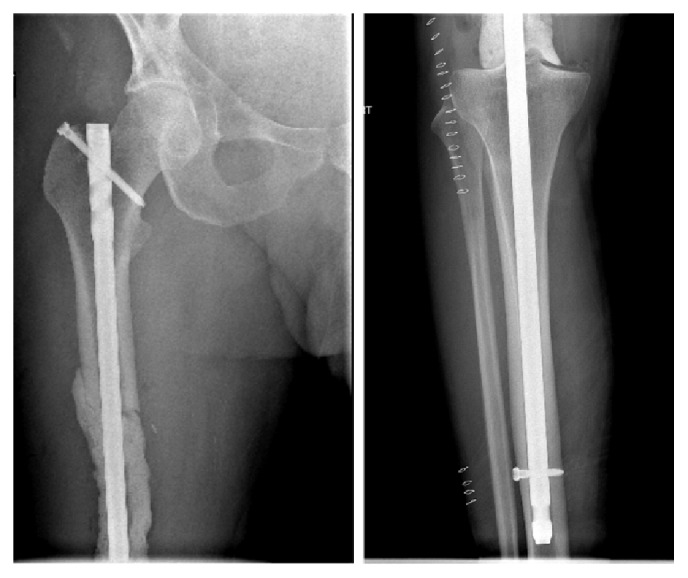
Postoperative AP radiographs showing long nail with surrounding cement.
